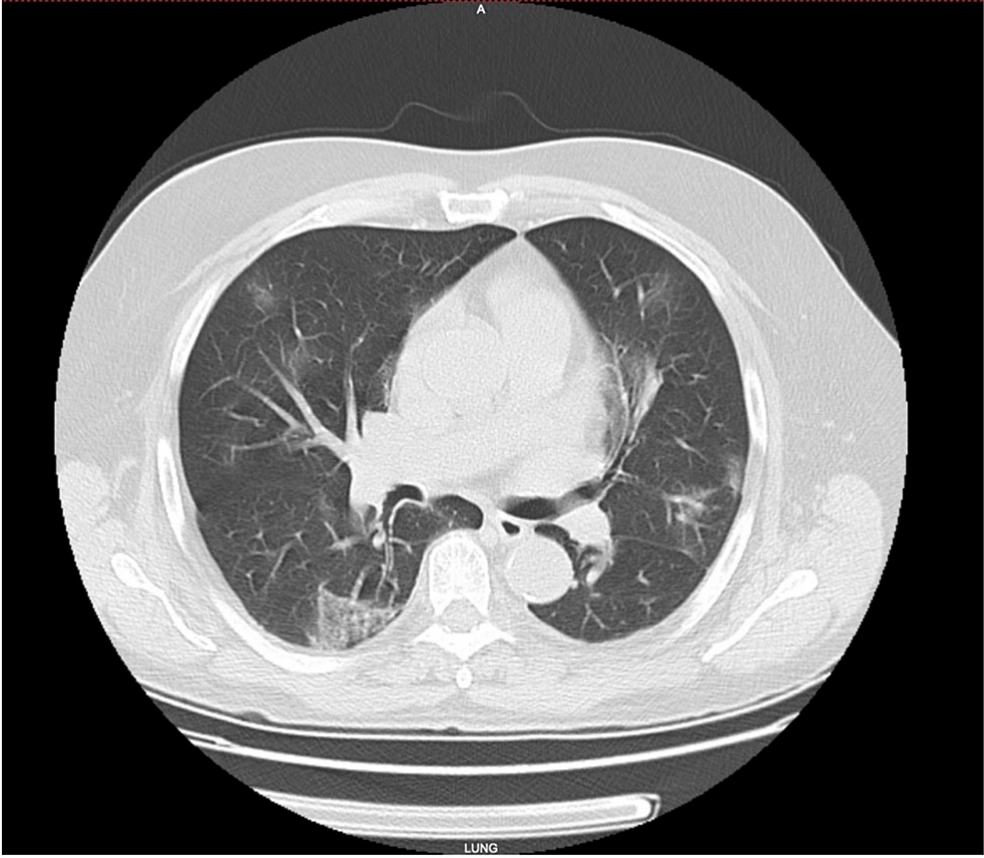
CT of the chest without IV contrast on arrival to our hospital depicting ground glass opacities consistent with a COVID-19 infection
